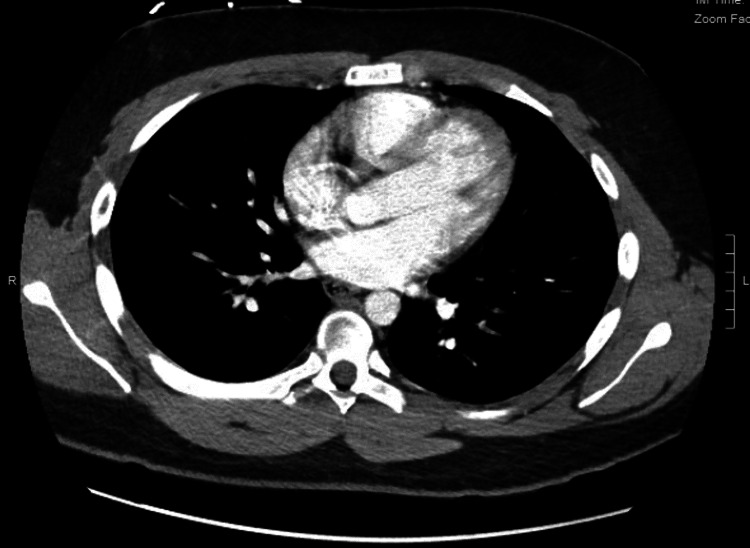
CT scan of the chest showing normal heart size. No pericardial effusion and unremarkable chest wall.
